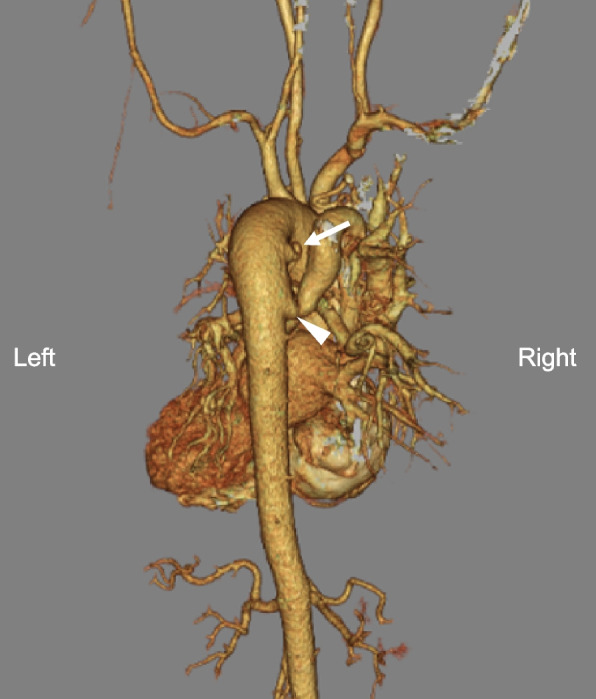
The 3D computed tomography shows that major aortopulmonary collateral arteries (MAPCAs) arise from descending aorta and course to bilateral pulmonary arteries. Upper MAPCA (arrow) courses to the left lung and the lower MAPCA (arrowhead) to the right lung
